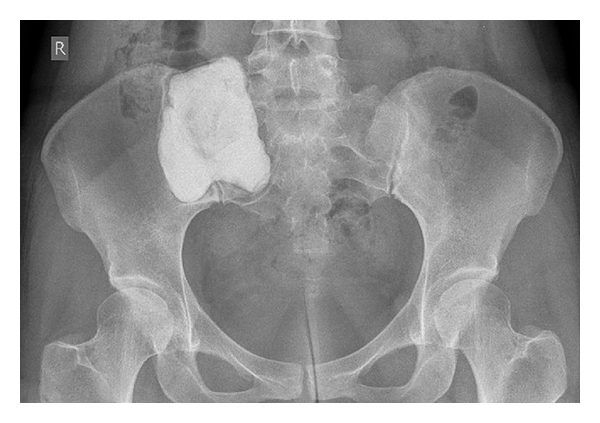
X-ray after curettage and bone cement packing of a sacral tumor. Postoperative X-ray of case 14 after intralesional curettage and bone cement packing.
